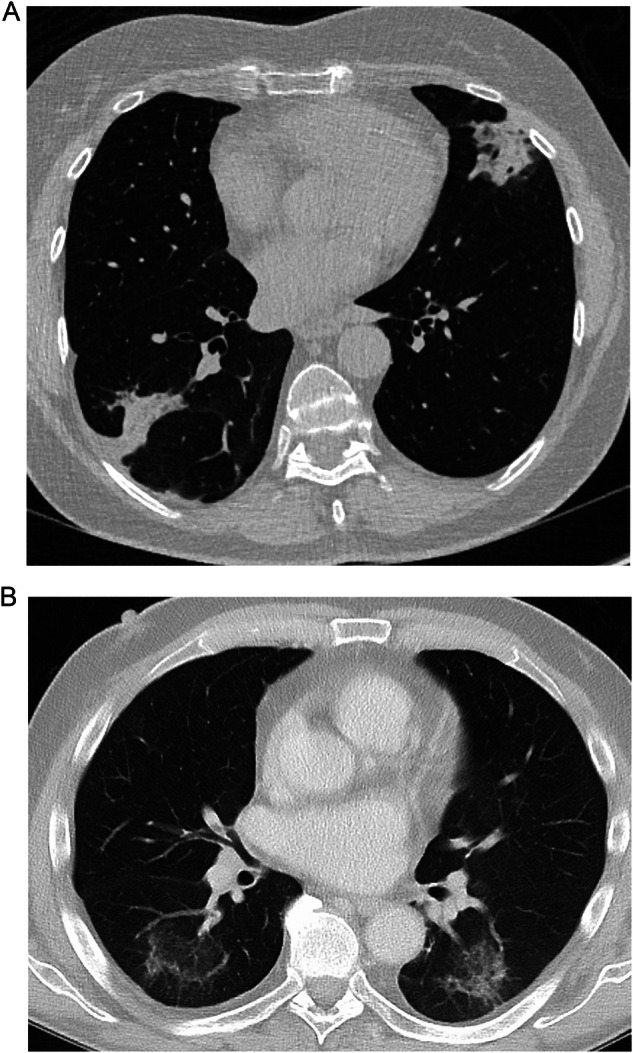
A CT scan of a patient with breast cancer and drug-induced ILD with OP pattern, characterized by sharply demarcated, bilateral, peripheral peribronchial consolidations (diagnosed by a multidisciplinary team [MDT] consensus); B CT scan of a patient with lung cancer and MDT-diagnosed drug-induced ILD with OP pattern and atoll sign in both under lobes. Images reproduced with permission from Dinkel J. 2023. University Hospital LMU Munich, Munich, Germany
